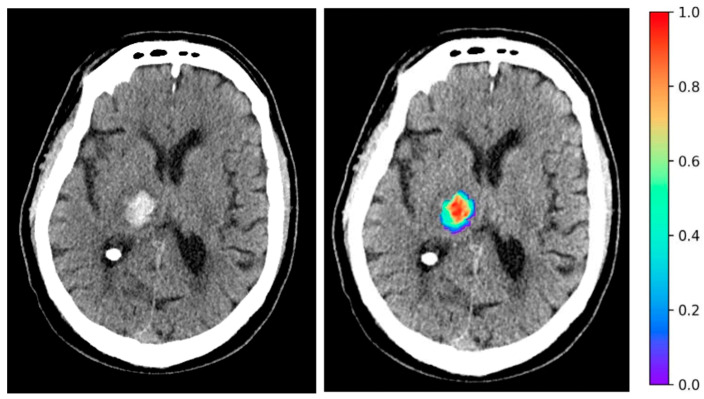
Example highlighting the ICH lesion original first-order energy radiomic feature on an axial slice of head CT scan.
